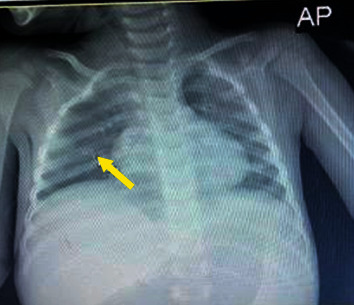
Chest radiography AP view.
